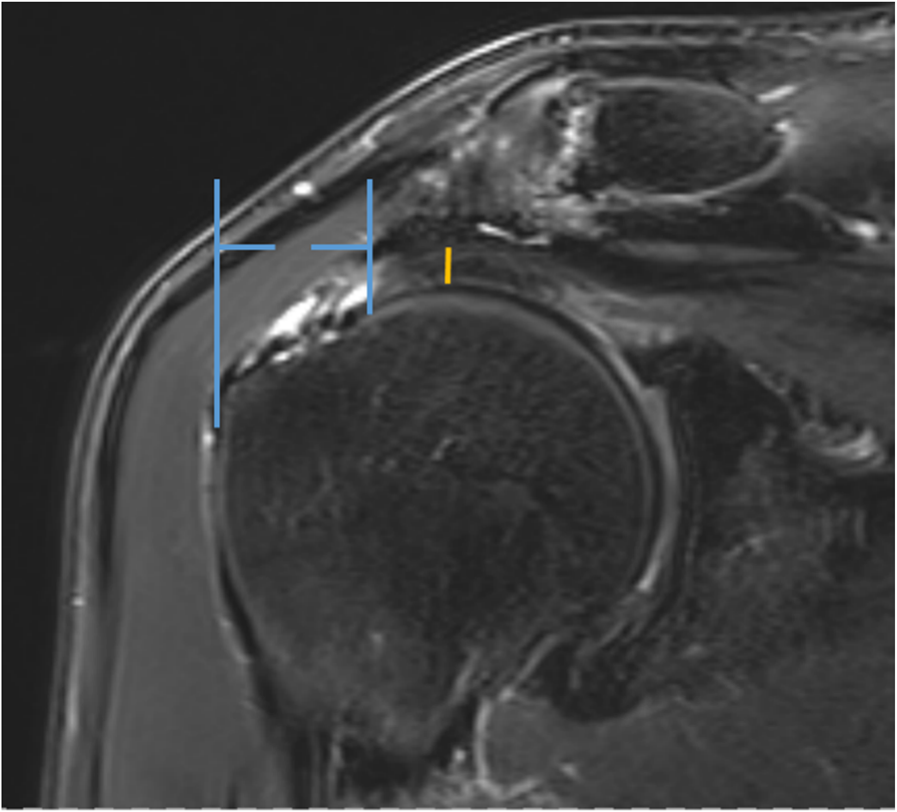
Tendon retraction and thickness were measured in the coronar plane of a t2-weighted MRI slice. Tendon retraction was measured between the lateral margin of the ruptured tendon and the lateral border of the supraspinatus foot print. Tendon thickness was measured at the thinnest portion of the tendon (yellow line)
